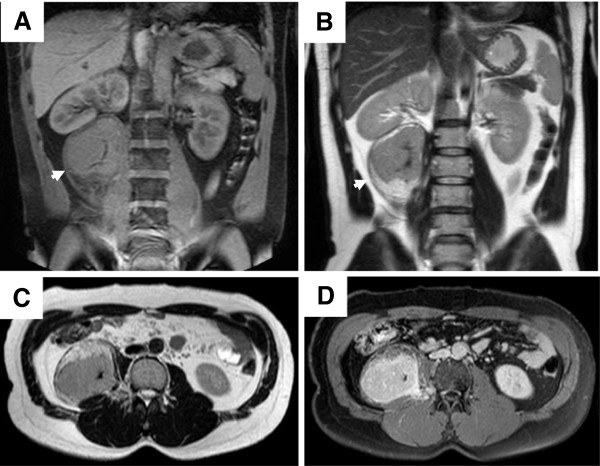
Magnetic resonance imaging. The mass presented isointensity on the T1-weighted image (A) and hyperintensity on the T2-weighted image (B) with a central radial hypointense area. On the caudal and anterior side of the tumor, a lipomatous component was observed on T2-weighted images (B,C). The contrast-enhanced image showed marked enhancement of the tumor (D).
